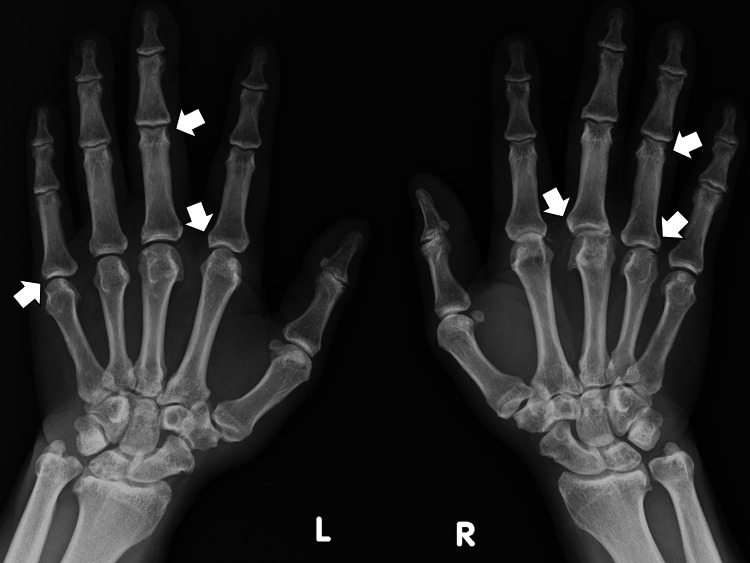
A hand X-ray showing several bone erosions in the affected joints (white arrows)
